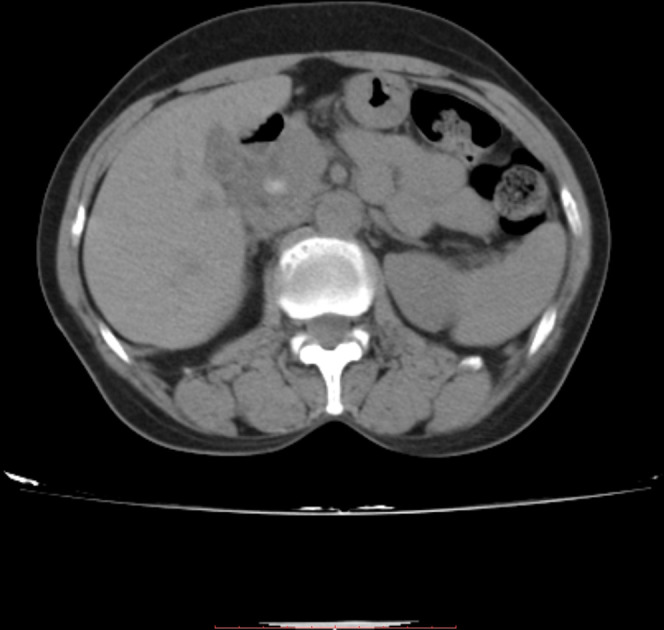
Non-contrast CT axial image showing calcified cystic duct calculus.
